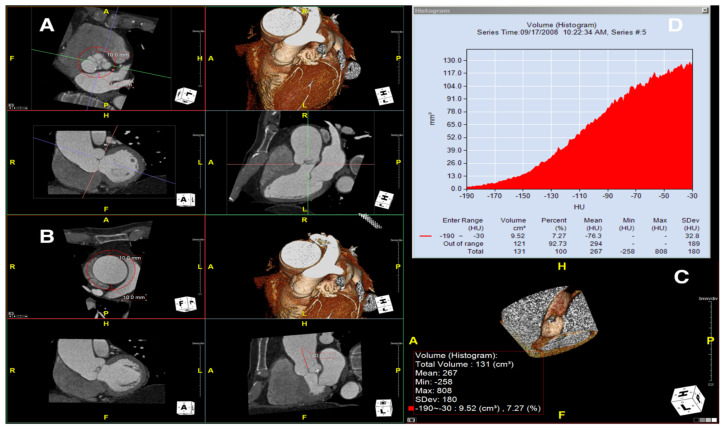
Step-by step process used to measure peri-aortic adipose tissue attenuation at Computed Tomography imaging. After spatial planes reorientation along the long and short axis of the ascending aorta, a 2D circle using an incremental +10 mm radius beyond the aortic diameter at the cusps level is traced (A) and a second circle is then drawn 40 mm distally, using in this case the diameter of the aorta at that level (B). The software automatically interpolates the volume between the two circles and builds the 3D volume sample (C), whose volume is known (131 cm^3^ in this case). The software also calculates the volume corresponding to a pre-determined Hounsfield Unit range; for fat sampling we used a −190 to −30 Hounsfield units (HU) range (in this case a volume of 9.52 cm^3^ is automatically measured). Panel (D) (fat attenuation histogram) shows that the mean attenuation of fat found in this custom sample volume shown in panel C is −76.3 HU.
